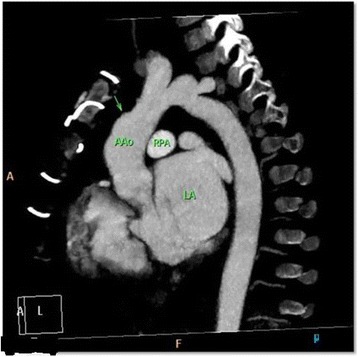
Computed tomography angiogram after surgery showed no aneurysm (arrow). *AAo* ascending aorta, *LA* left atrium, *RPA* right pulmonary artery
